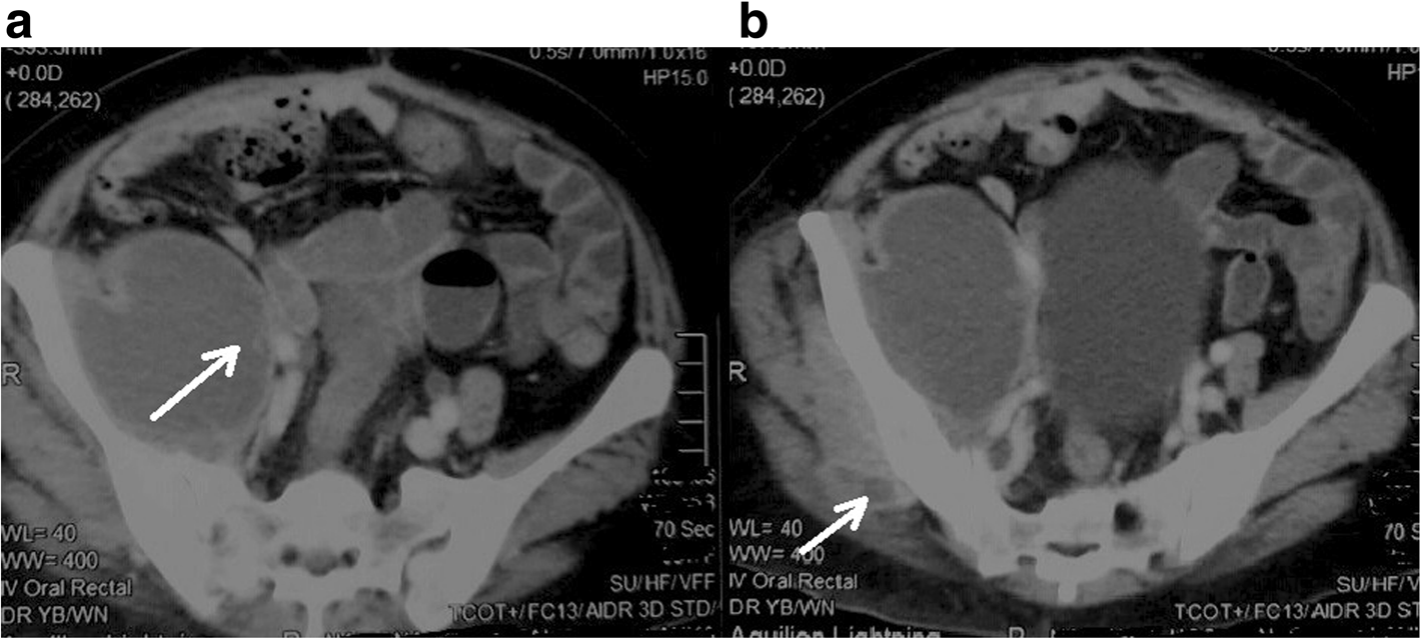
a and b Axial view of Contrast Enhanced CT Abdomen and pelvis images showing cystic lesion with an enhancing wall suggesting abscesses in right psoas, iliacus muscles extending to gluteus muscles(white arrows)
